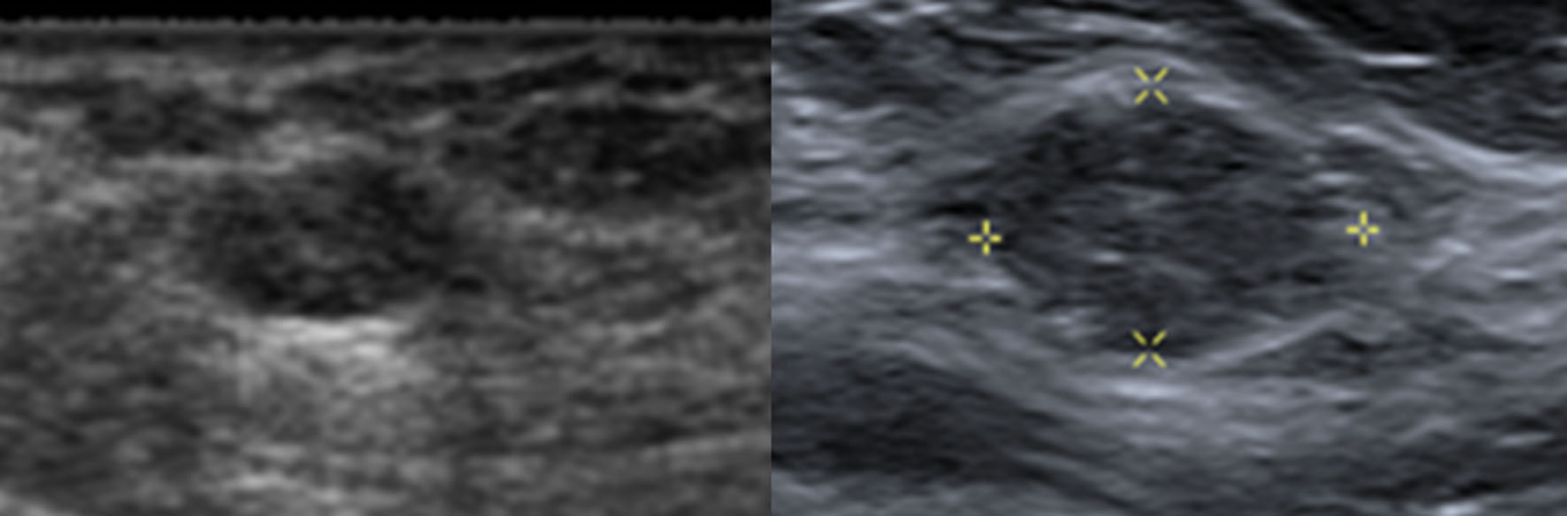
Interval growth of a BI-RADS 3 mass. This solid oval mass on ultrasound was assessed as BI-RADS 3. However, patient was lost to follow up and did not return for 2 years. The mass was biopsied at 2-year follow-up due to interval growth yielding mucinous carcinoma
